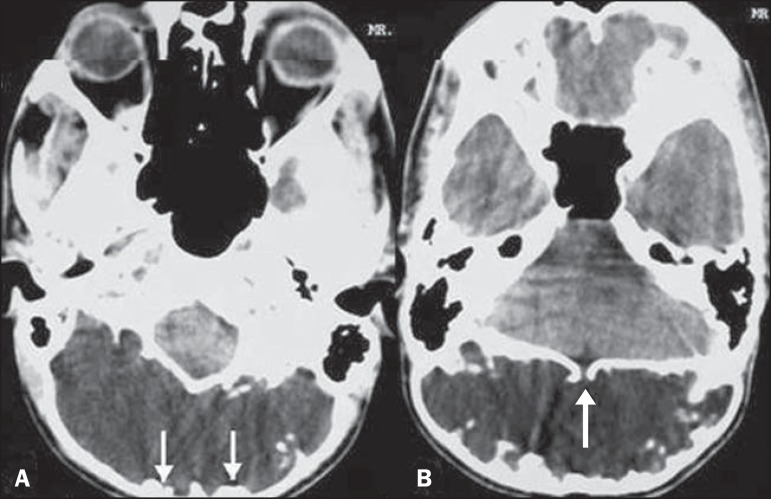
A: Non-contrast-enhanced CT scan of the head showing a large
